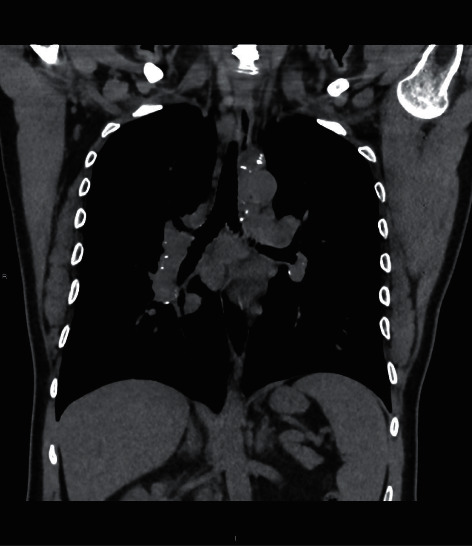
Chest CT: mediastinal and hilar adenopathy with coarse eggshell classification.
